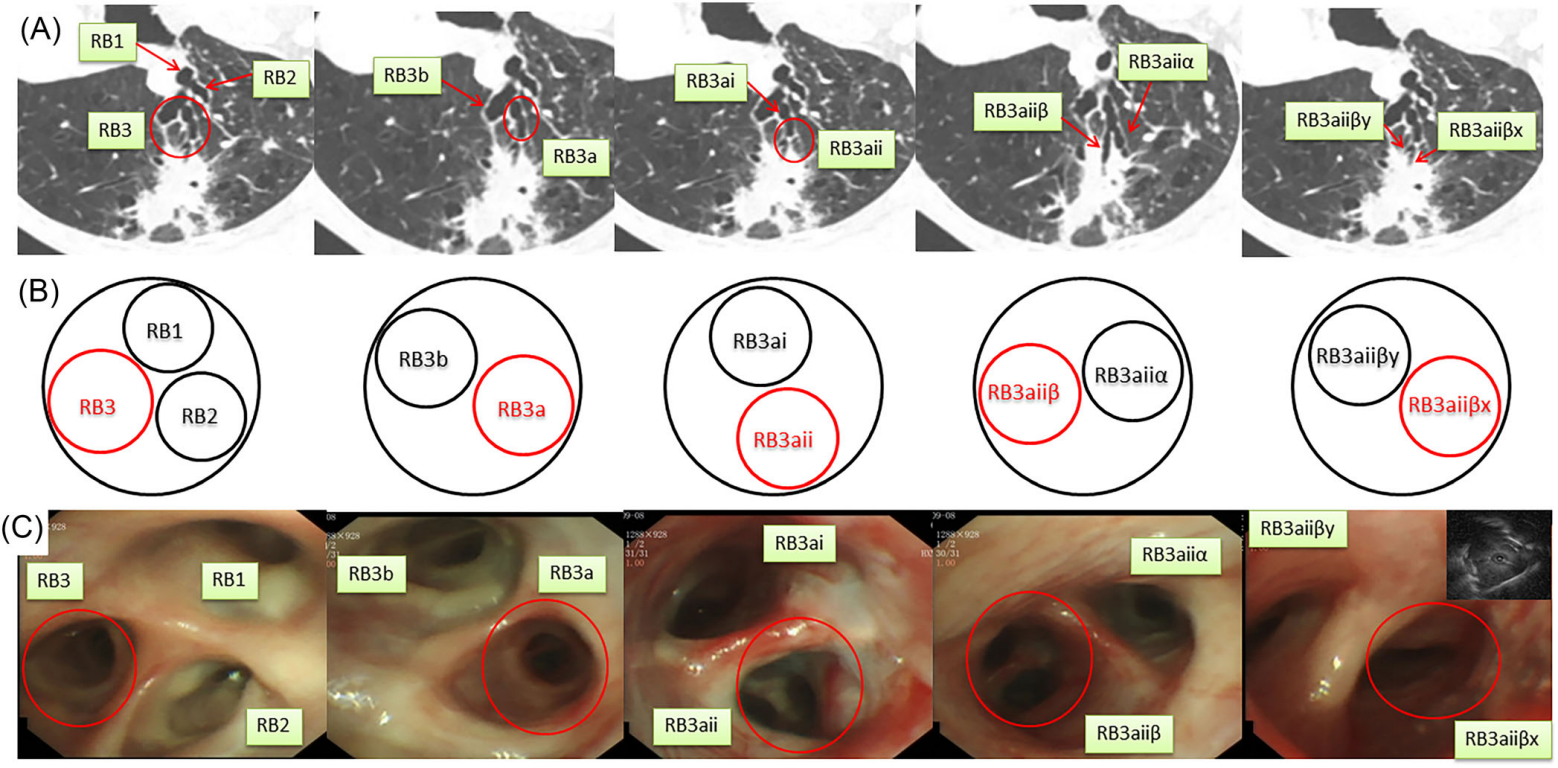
A representative case of right upper lobe lesion. (A) Rolled the computed tomography images counterclockwise 90° to correlate with the orientation in bronchoscopic view. (B) Drew the sketches at every bronchial bifurcation at precisely the same angle with the rotated computed tomography showed. (C) The sketches were exactly the same as the actual bronchoscopic views, and within radial endobronchial ultrasound image was identified at target RB3aiiβx. This patient was diagnosed as tuberculosis by radial endobronchial ultrasound bronchoscopy.
